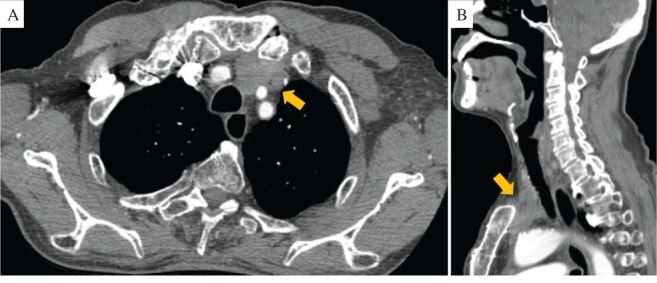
Chest computed tomography (CT) showed a tumour in anterior mediastinum and the mass had remained unchanged over the past decade as determined on serial chest CT examinations.
